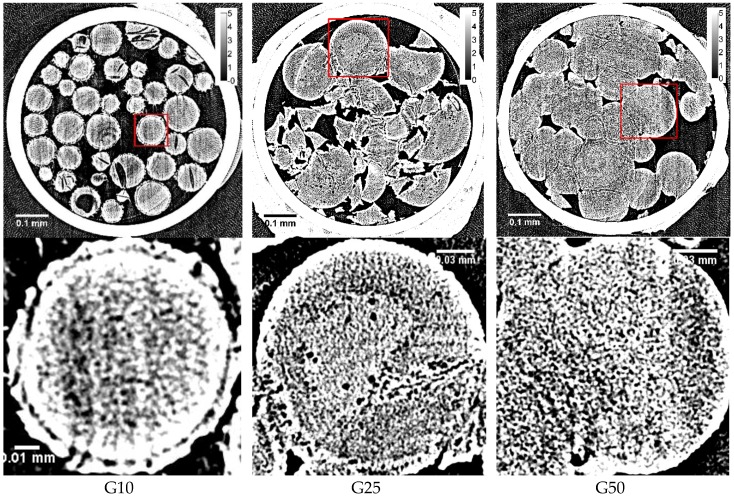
X-ray CT images obtained with slowly cooled Sephadex^®^ beads containing 50 wt % water (top) together with the expanded images of the square part of the corresponding upper ones (bottom): (left) G10; (middle) G25; (right) G50. Calibration bars on the upper right indicate the LAC (cm^−1^).
